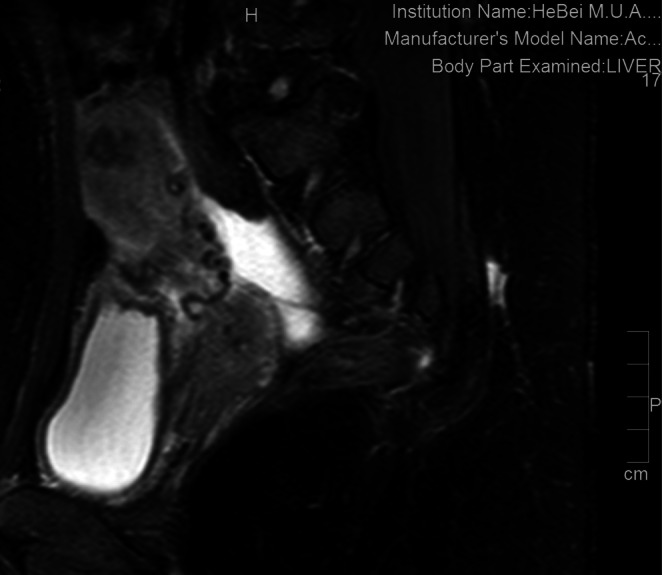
Imaging findings of the pelvis examined by MRI before treatment.
